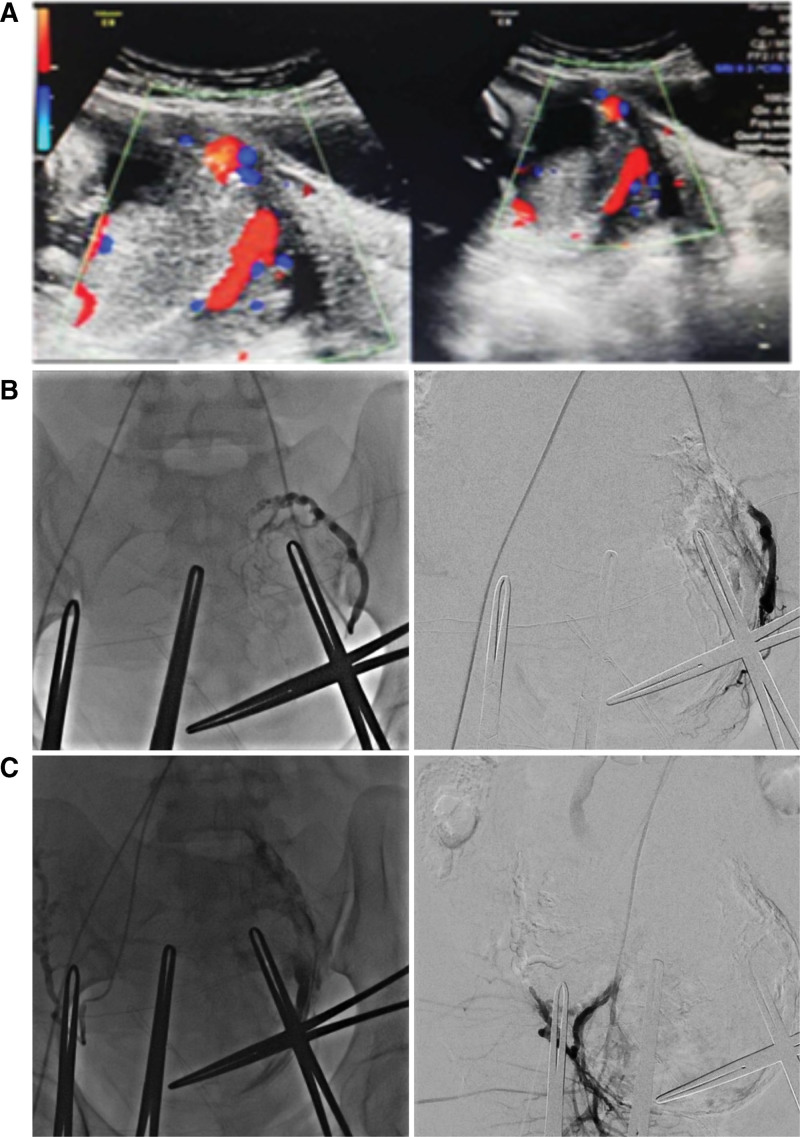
Placenta previa diagnosed by ultrasonography and effects of uterine artery embolization on the circulation of uterine arteries. (A) showed placenta completely covering cervical OS. (B) left (left uterine artery), (C) left (right uterine artery) showed the bilateral uterine arteries blood flow before uterine artery embolization. (B) right (left uterine artery), (D) right (right uterine artery) presented the bilateral uterine arteries blood flow after uterine artery embolization.
